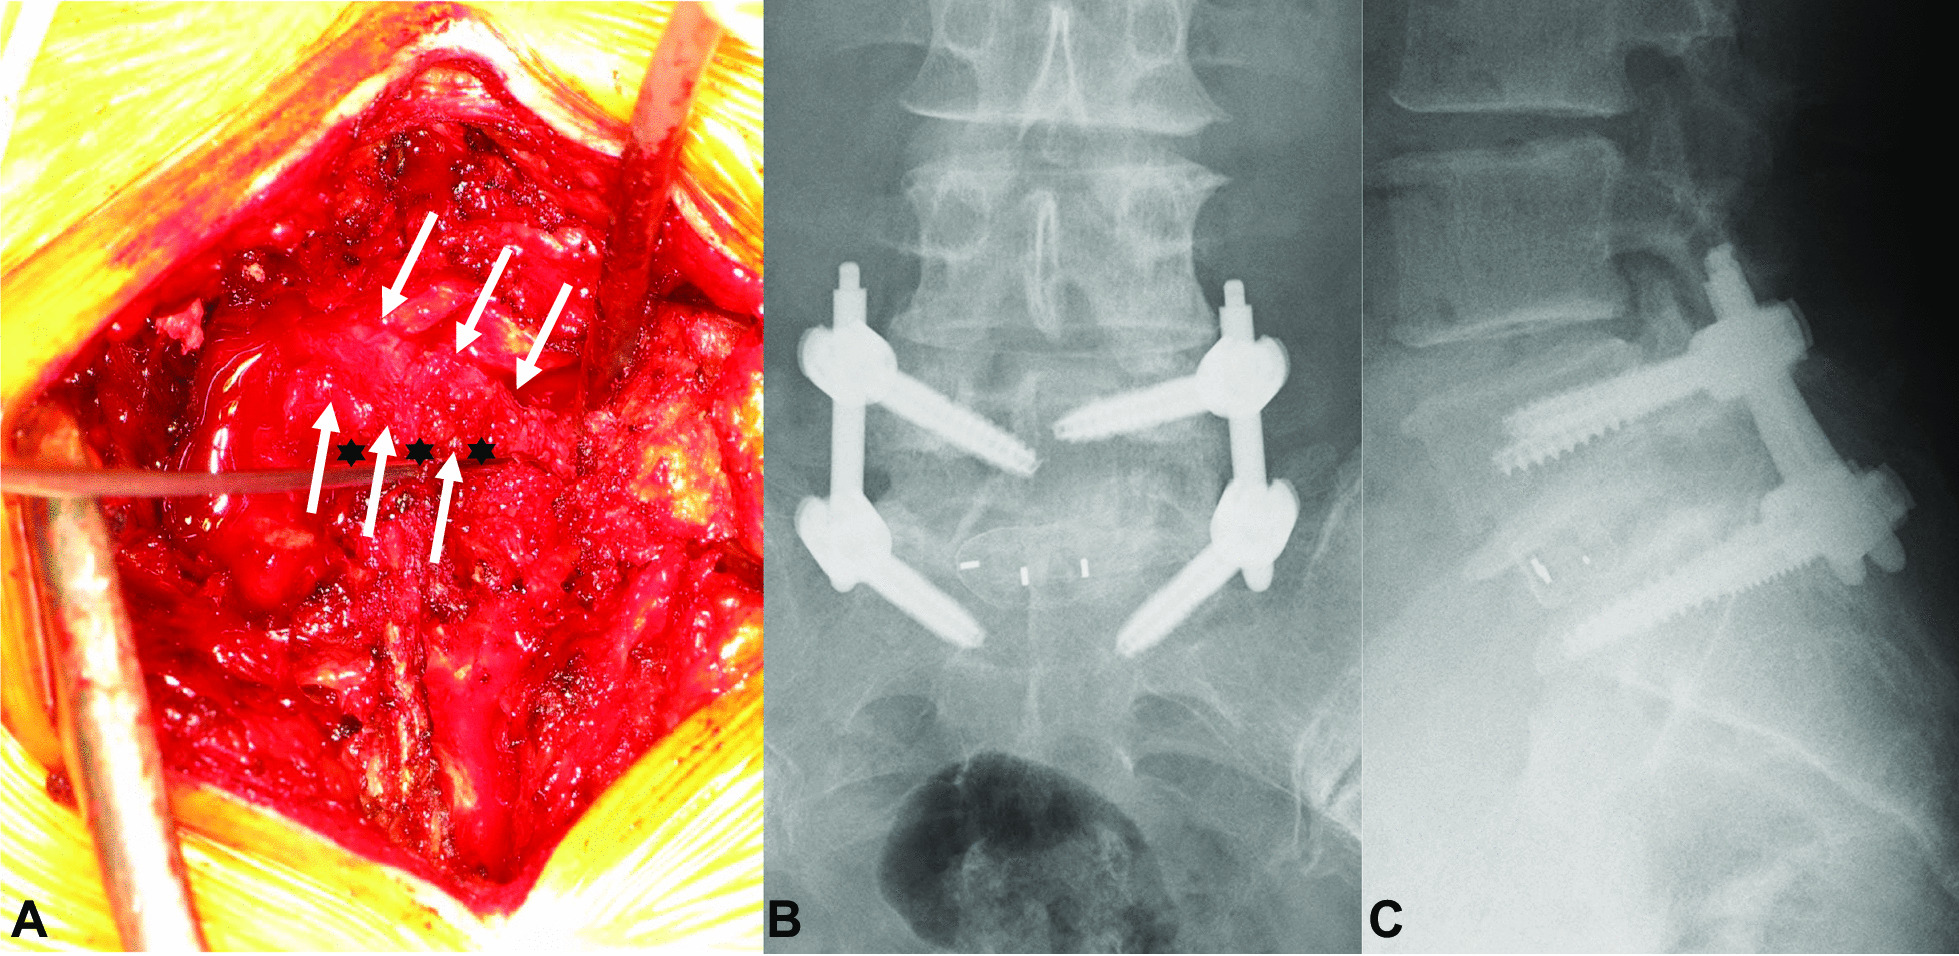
Right CNR (white arrow) crossing the L5–S disc space (heavy six-pointed black star). The right CNR is markedly swollen and cannot be safely mobilized (a intraoperative findings). Postoperative X-ray demonstrates successful L5–S TLIF (b AP view; c lateral view). *CNR* conjoined nerve root;* TLIF* transforaminal lumbar interbody fusion
